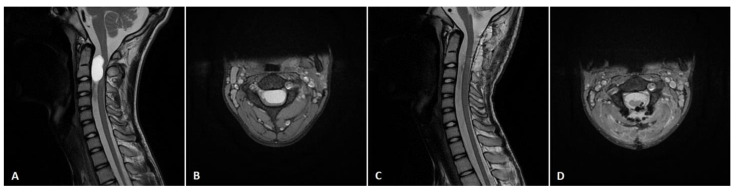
An exemplary case of a 14-year-old boy (patient no. 16), who presented with a left-sided motor deficit in the upper limb. Preoperative T2 (A) sagittal and (B) axial MRI scan showed C1-C2 intradural extramedullary tumor. The patient underwent gross-total tumor resection via the posterior approach with laminectomy. Postoperative T2 (C) sagittal and (D) axial MRI scan confirmed complete tumor removal. Postoperatively, patients’ motor function improved.
